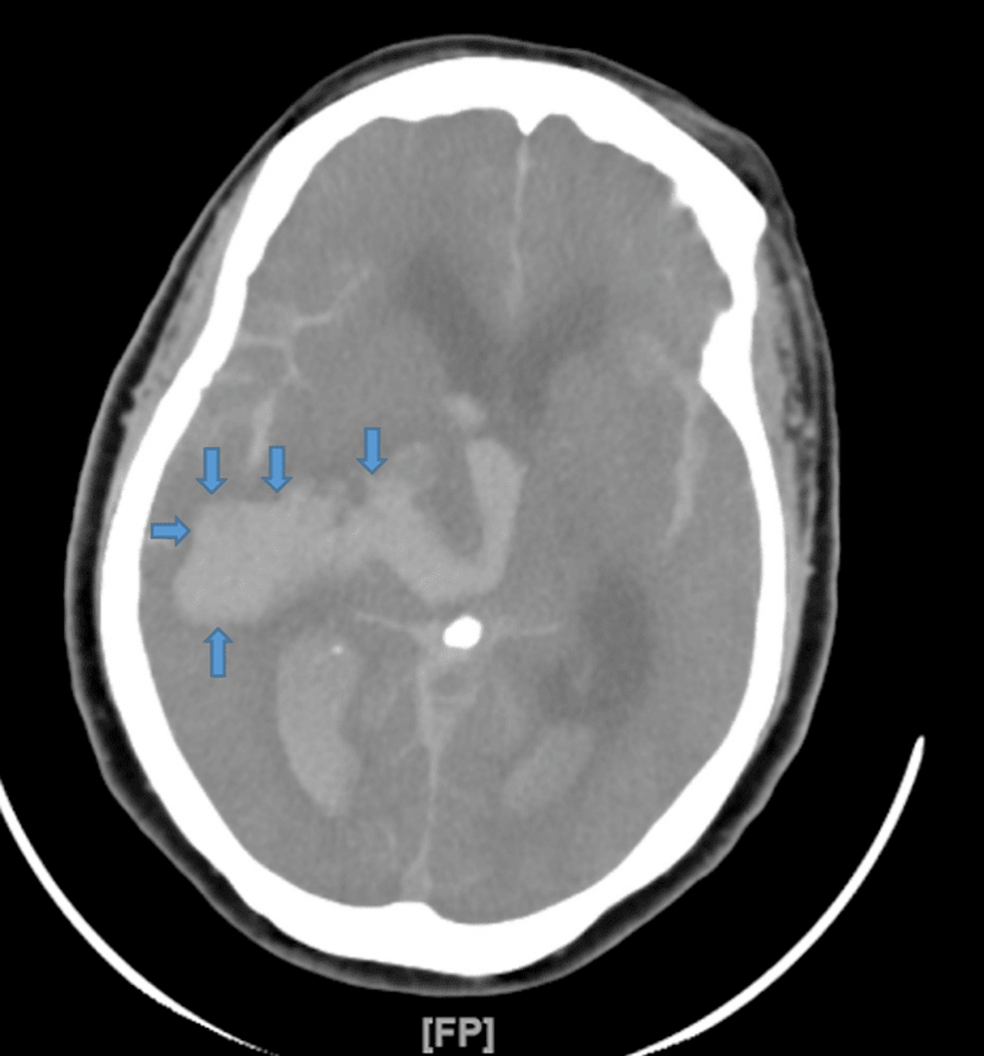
Computed tomography scan of the head without contrast revealing massive acute intracranial hemorrhage.
